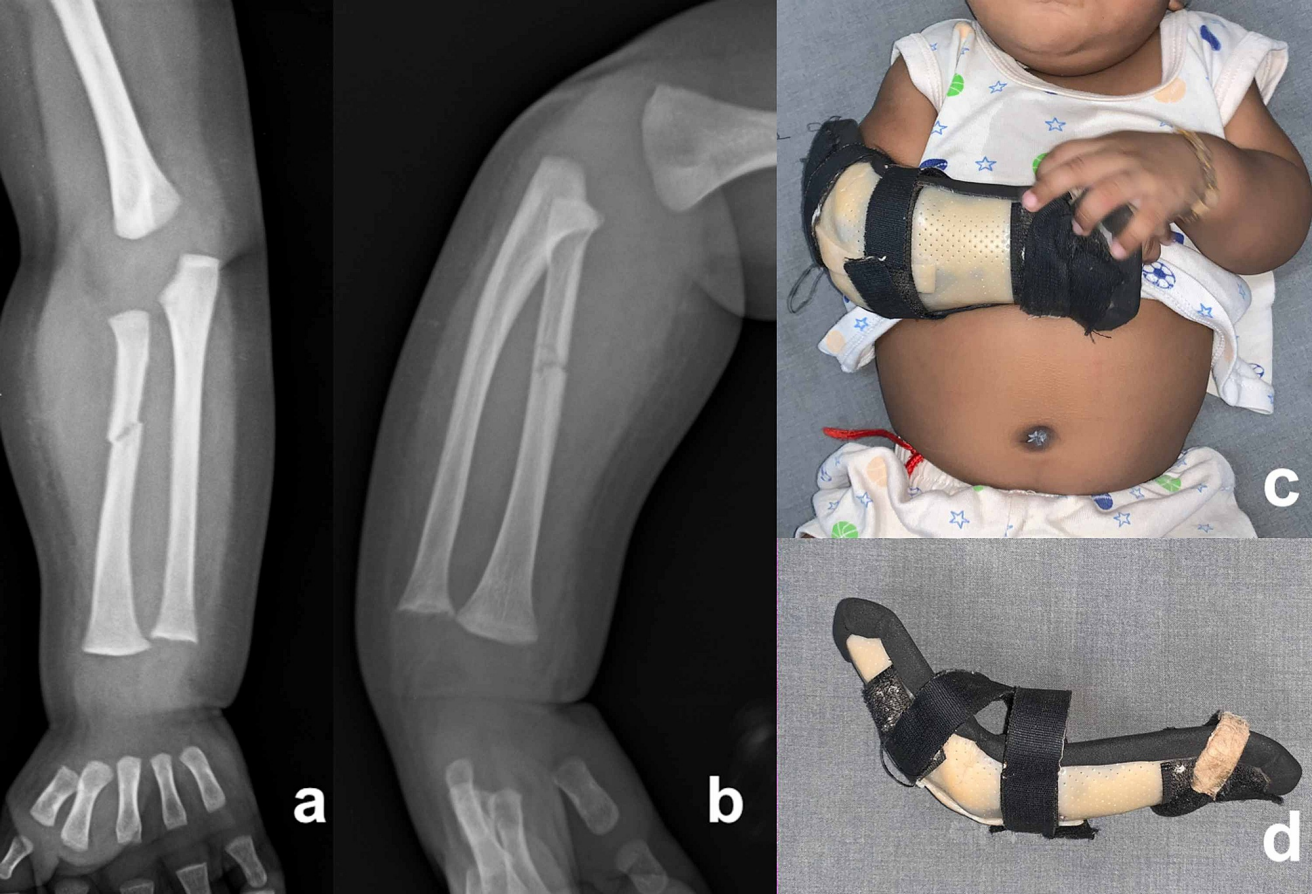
Radiographs at the time of diagnosis Radiographs of a two-month-old boy show isolated radius shaft fracture in posteroanterior (a) and oblique views (b). Clinical pictures of the boy (c) with an above-elbow thermoplastic splint (d) two weeks after the injury
